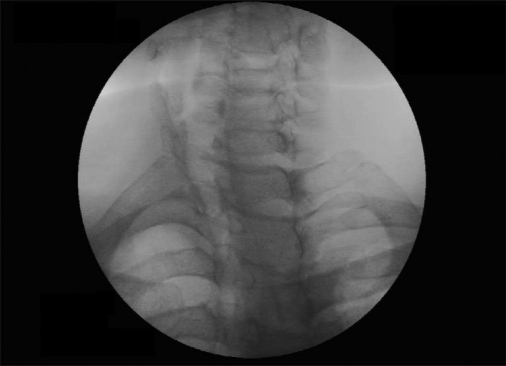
X-ray image shows uncinate process of C7 and intervertebral foramina
